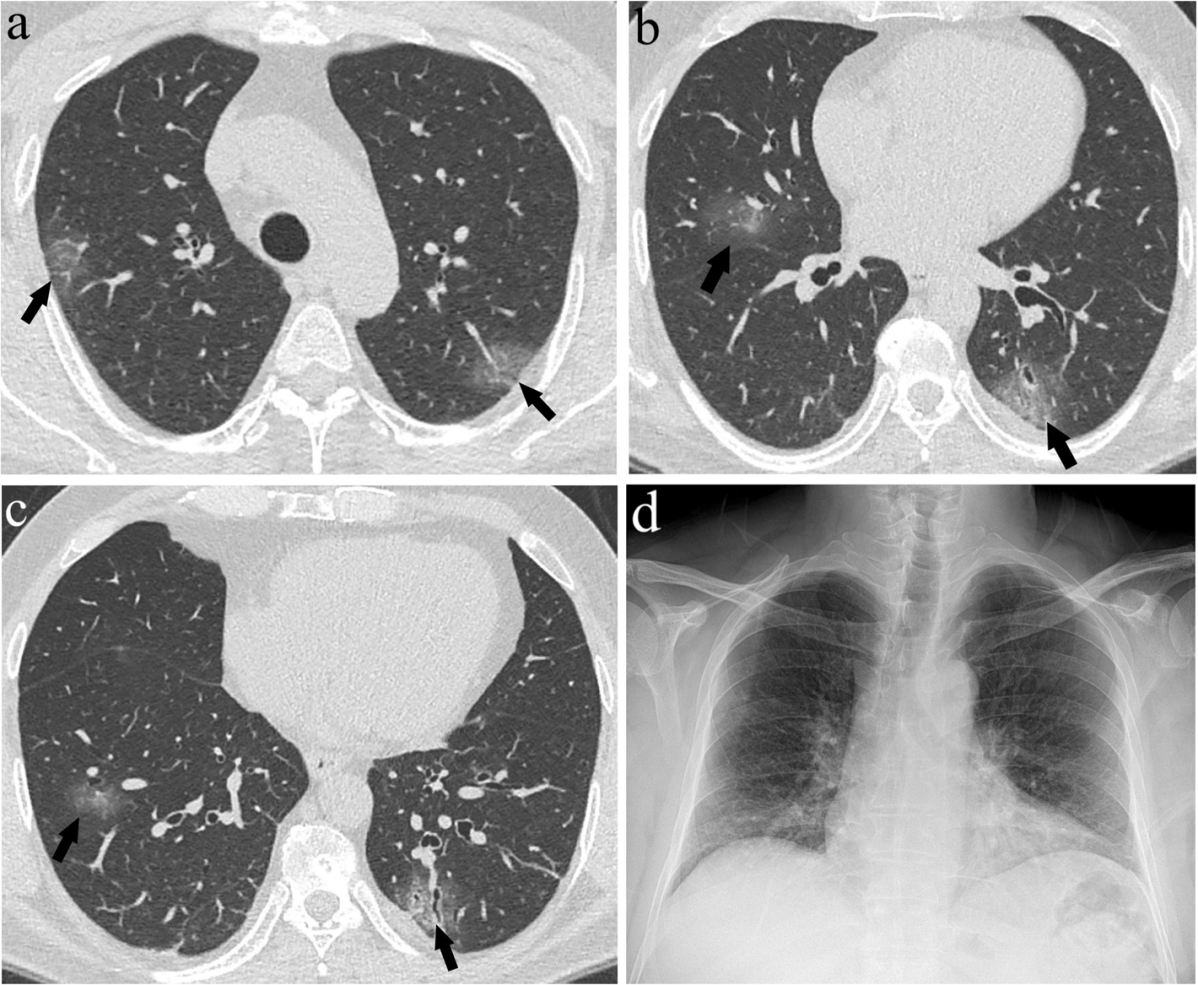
a–d A 56-year-old male presenting with dry cough and fever for 3 days. Axial CT images at the level of the upper (a), middle (b), and lower (c) lung zones demonstrate ground glass opacities representing grade 1 lesions (arrows) (CTS = 6). There were no signs of pneumonia on the CXR image (d)
